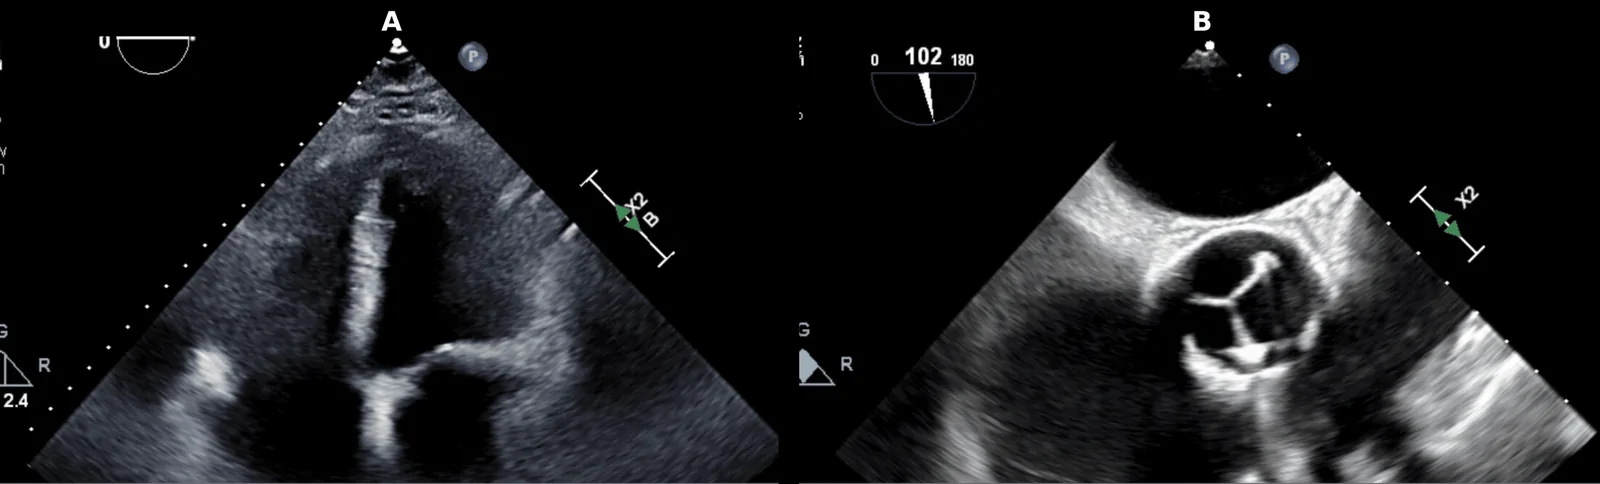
Imaging findings (first admission) Transthoracic (A) and transesophageal (B) echocardiography, demonstrating the bioprosthetic aortic valve without demonstrable vegetation, abscess, or periannular complication.
